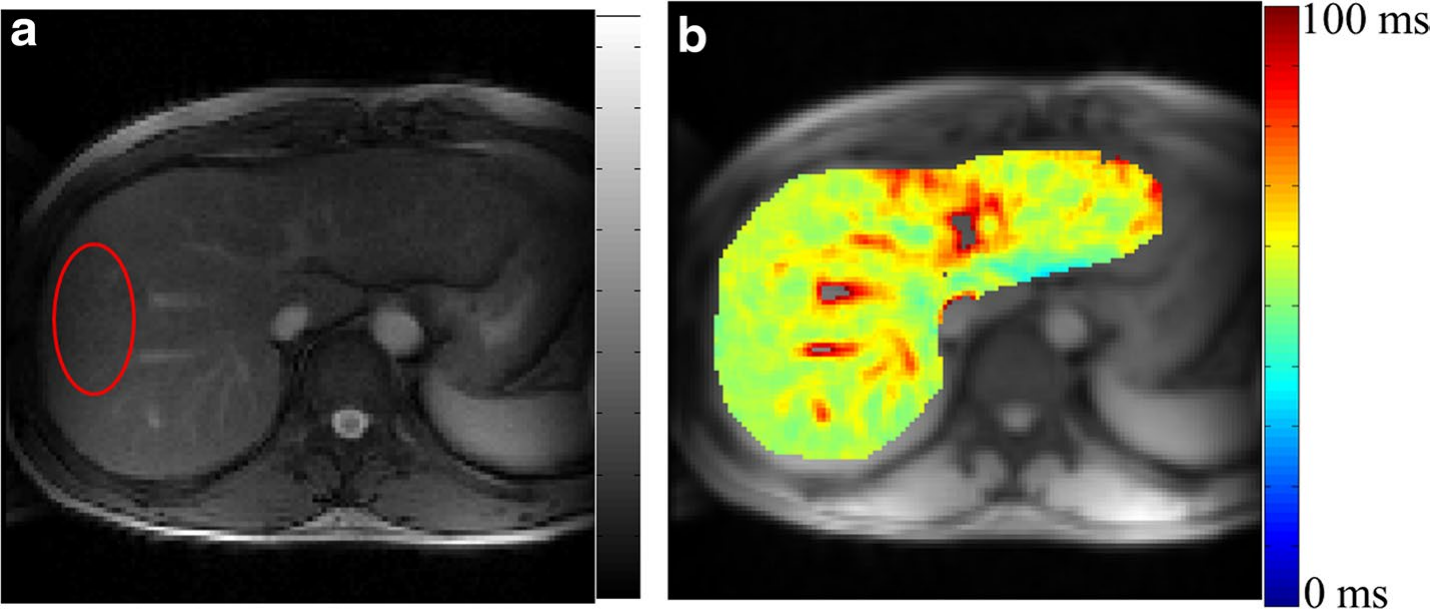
T1ρ MRI weighted image corresponding to TSL = 30 ms (a) and map (b) of healthy human liver. T1ρ map of segmented liver is color overlaid on anatomical image. T1ρ value in ROI marked on weighted image (a) is 55.6 ± 2.3 ms
